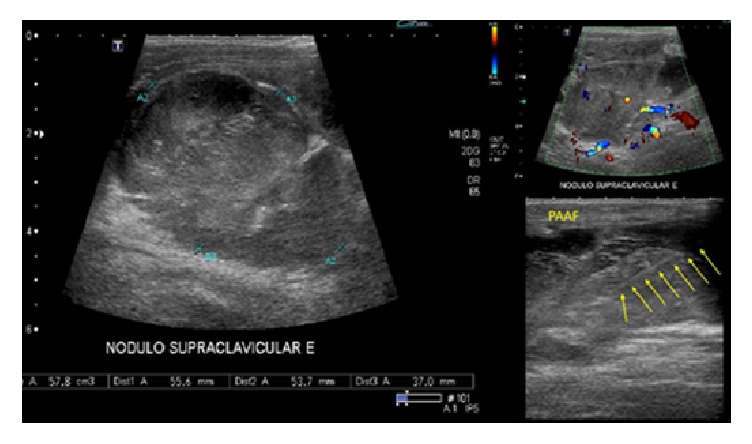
Ultrasonography of the neck demonstrating the shape and vascularization of left supraclavicular lymphadenopathy. It also demonstrates the puncture/biopsy by fine needle of the nodule (FNAB), highlighted by the yellow arrows.
